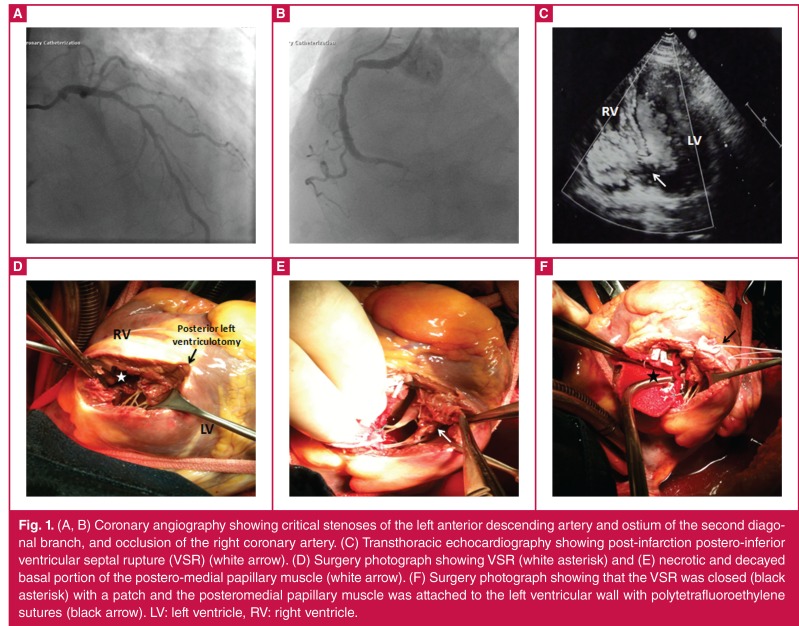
(A, B) Coronary angiography showing critical stenoses of the left anterior descending artery and ostium of the second diagonal branch, and occlusion of the right coronary artery. (C) Transthoracic echocardiography showing post-infarction postero-inferior ventricular septal rupture (VSR) (white arrow). (D) Surgery photograph showing VSR (white asterisk) and (E) necrotic and decayed basal portion of the postero-medial papillary muscle (white arrow). (F) Surgery photograph showing that the VSR was closed (black asterisk) with a patch and the posteromedial papillary muscle was attached to the left ventricular wall with polytetrafluoroethylene sutures (black arrow). LV: left ventricle, RV: right ventricle.
